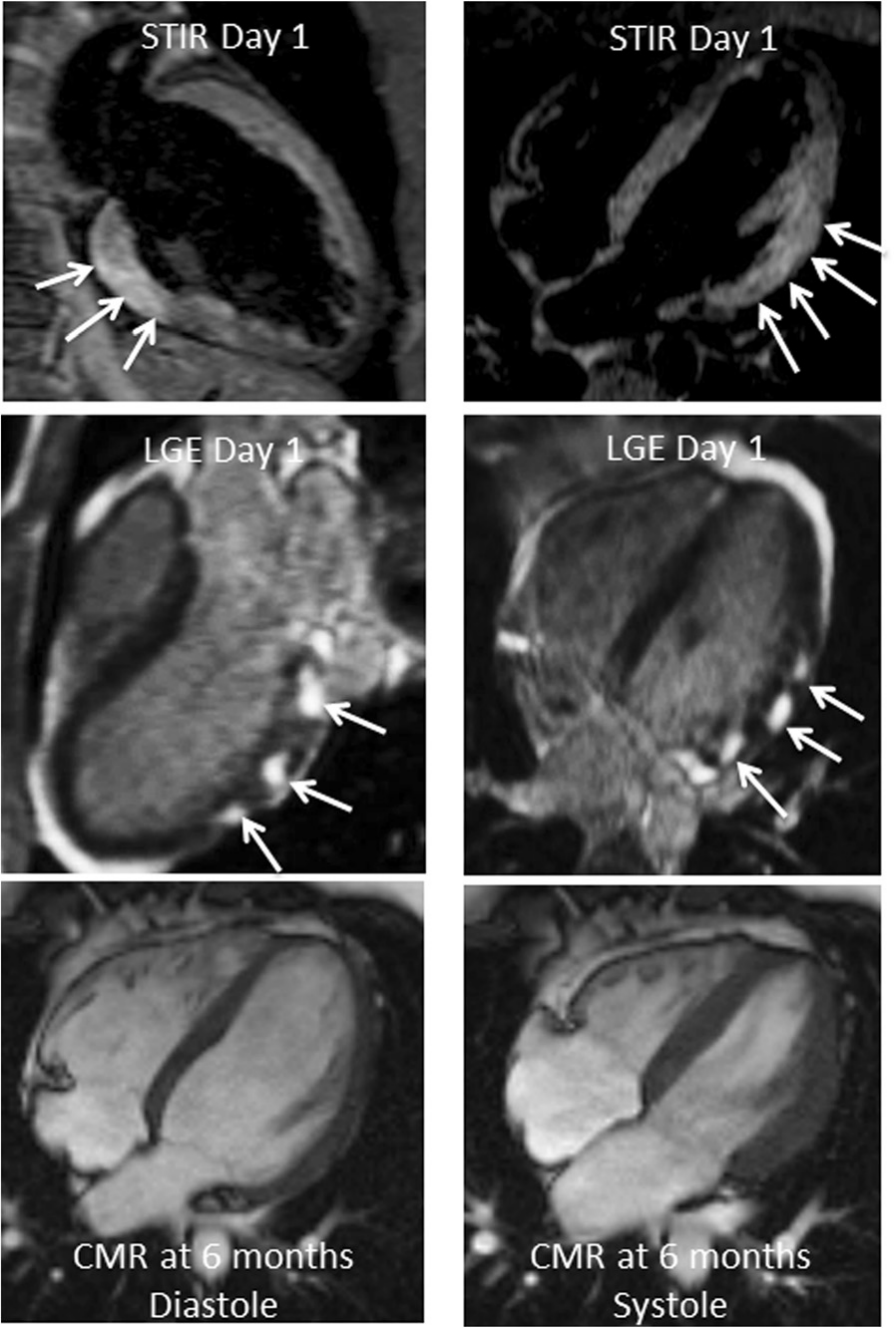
CMR data of a 25 year-old male performed at day 1 after the onset of an acute coronary-like syndrome (chest pain, ST segment alterations, mild troponin elevation). Black blood T2-weighted STIR CMR indicates the presence of segmental diffuse myocardial edema of the LV posterior and lateral walls representing 23 % of LV mass (top panel, arrows). Late gadolinium-enhanced CMR shows diffuse subepicardial nodular lesions in the posterolateral and lateral walls of the LV (11 % of LV mass) indicative of acute myocarditis (mid panel, arrows). Cine-MR at day 1 and 6 months (bottom panel) showed normal systolic global and segmental LV function (LV end-diastolic volume index 71 ml/m^2^, LV ejection fraction 61 %; 69 ml/m^2^ and 63 %, respectively). The patient did well and had no MACE during follow-up
